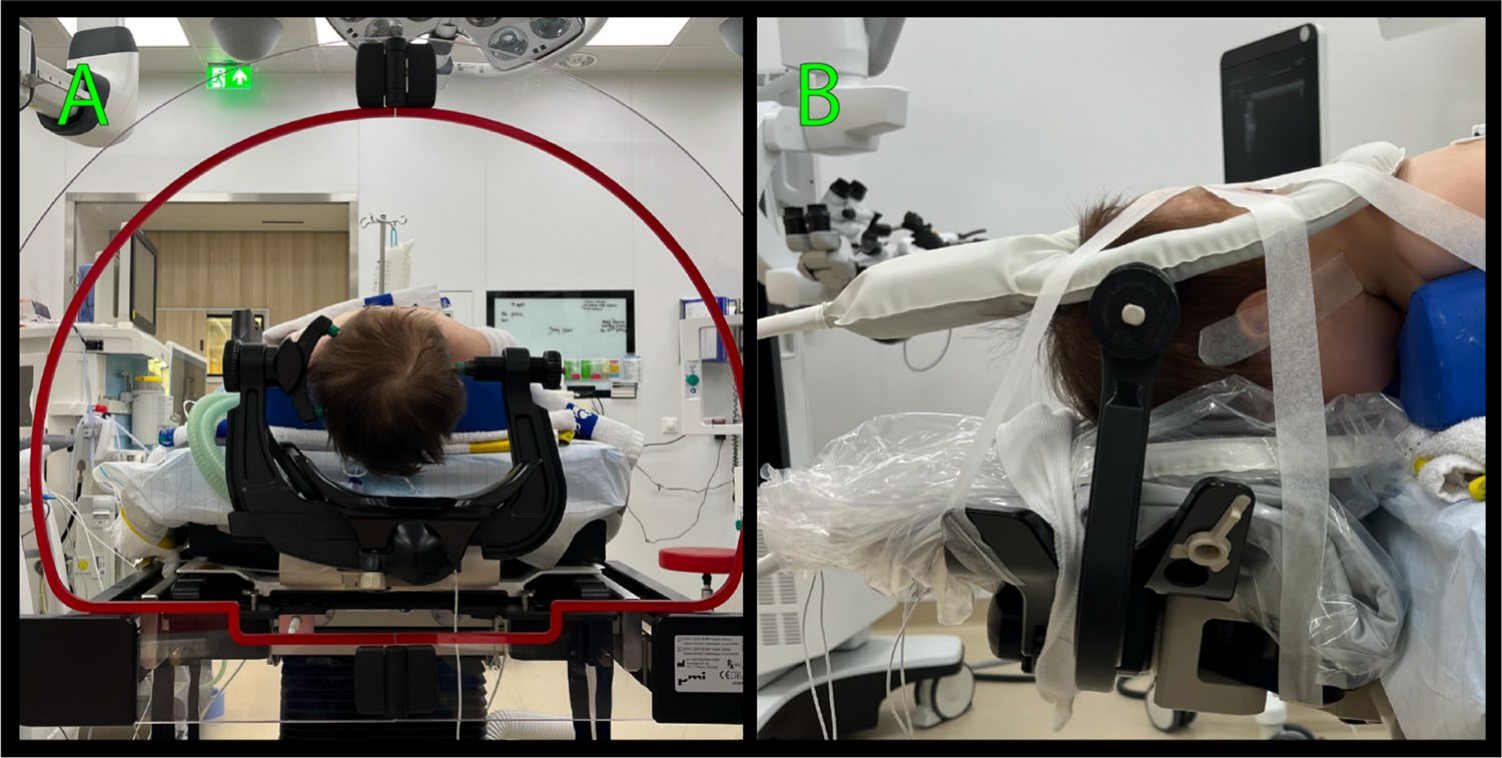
Example of patient preparation for MR acquisition before surgery. A After positioning the patient in the surgical prone position (i.e., laying on their stomach with their chest lifted and chin-down), the head is secured in the DORO surgical head-frame. B The two RF coils are then placed anterior and posterior of the patients’ head and fixated with tape before sliding the patient into the intraoperative MRI scanner that is in a room adjacent to the operation theater
